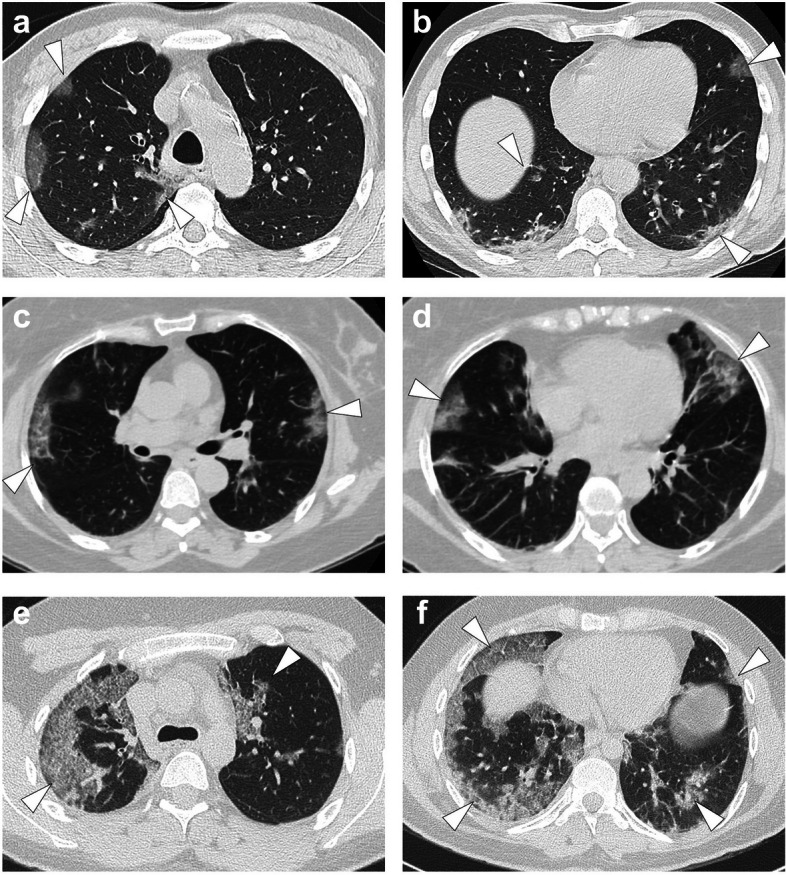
Differential diagnoses identified with the patients’ level analysis before February 2020. a, b Fifty-one years old man with kidney transplant addressed to the emergency for couch, dyspnoea and inflammatory syndrome in September, 2019. Final diagnosis was influenza A virus. c, d Fifty years old woman without significant medical history addressed to the emergency for flu syndrome, dyspnoea and low oxygen saturation (92%). Final diagnosis was influenza A virus. e, f Twenty-six years old male with dyspnoea, cough, inflammatory syndrome and low oxygen saturation (93.5%), already treated with 4 days of amoxicillin. Final diagnosis was influenza A virus and Mycoplasma pneumonia co-infection. All CT images are in axial plan and lung kernel. They showed bilateral subpleural ground glass opacities (white arrowheads) possibly combined with intralobular reticulations (providing a crazy paving pattern) and band-like condensations
